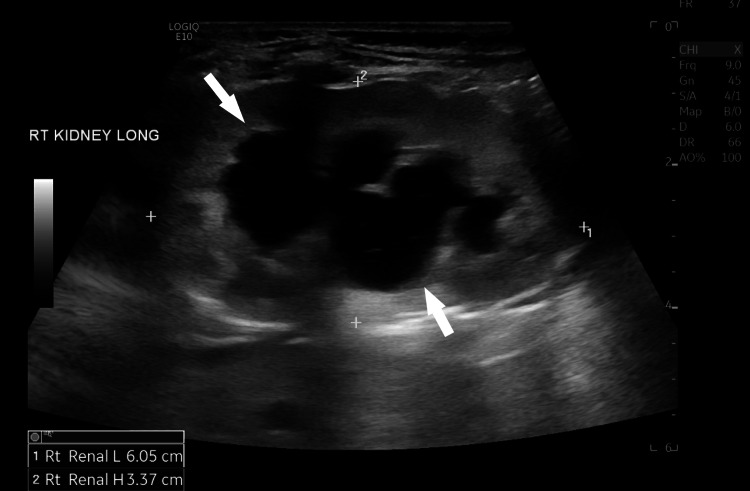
Renal ultrasound showing moderate right hydronephrosis
